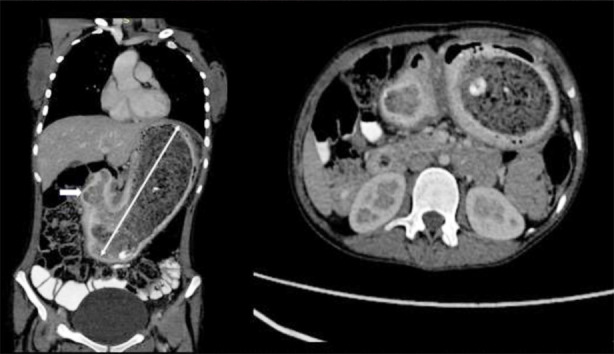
CT scan of the abdomen with contrast, coronal and axial sections: showing a large mass of heterogeneous material in the stomach, extending through the pylorus into the duodenum suggestive of a large trichobezoar (Rapunzel Syndrome).
